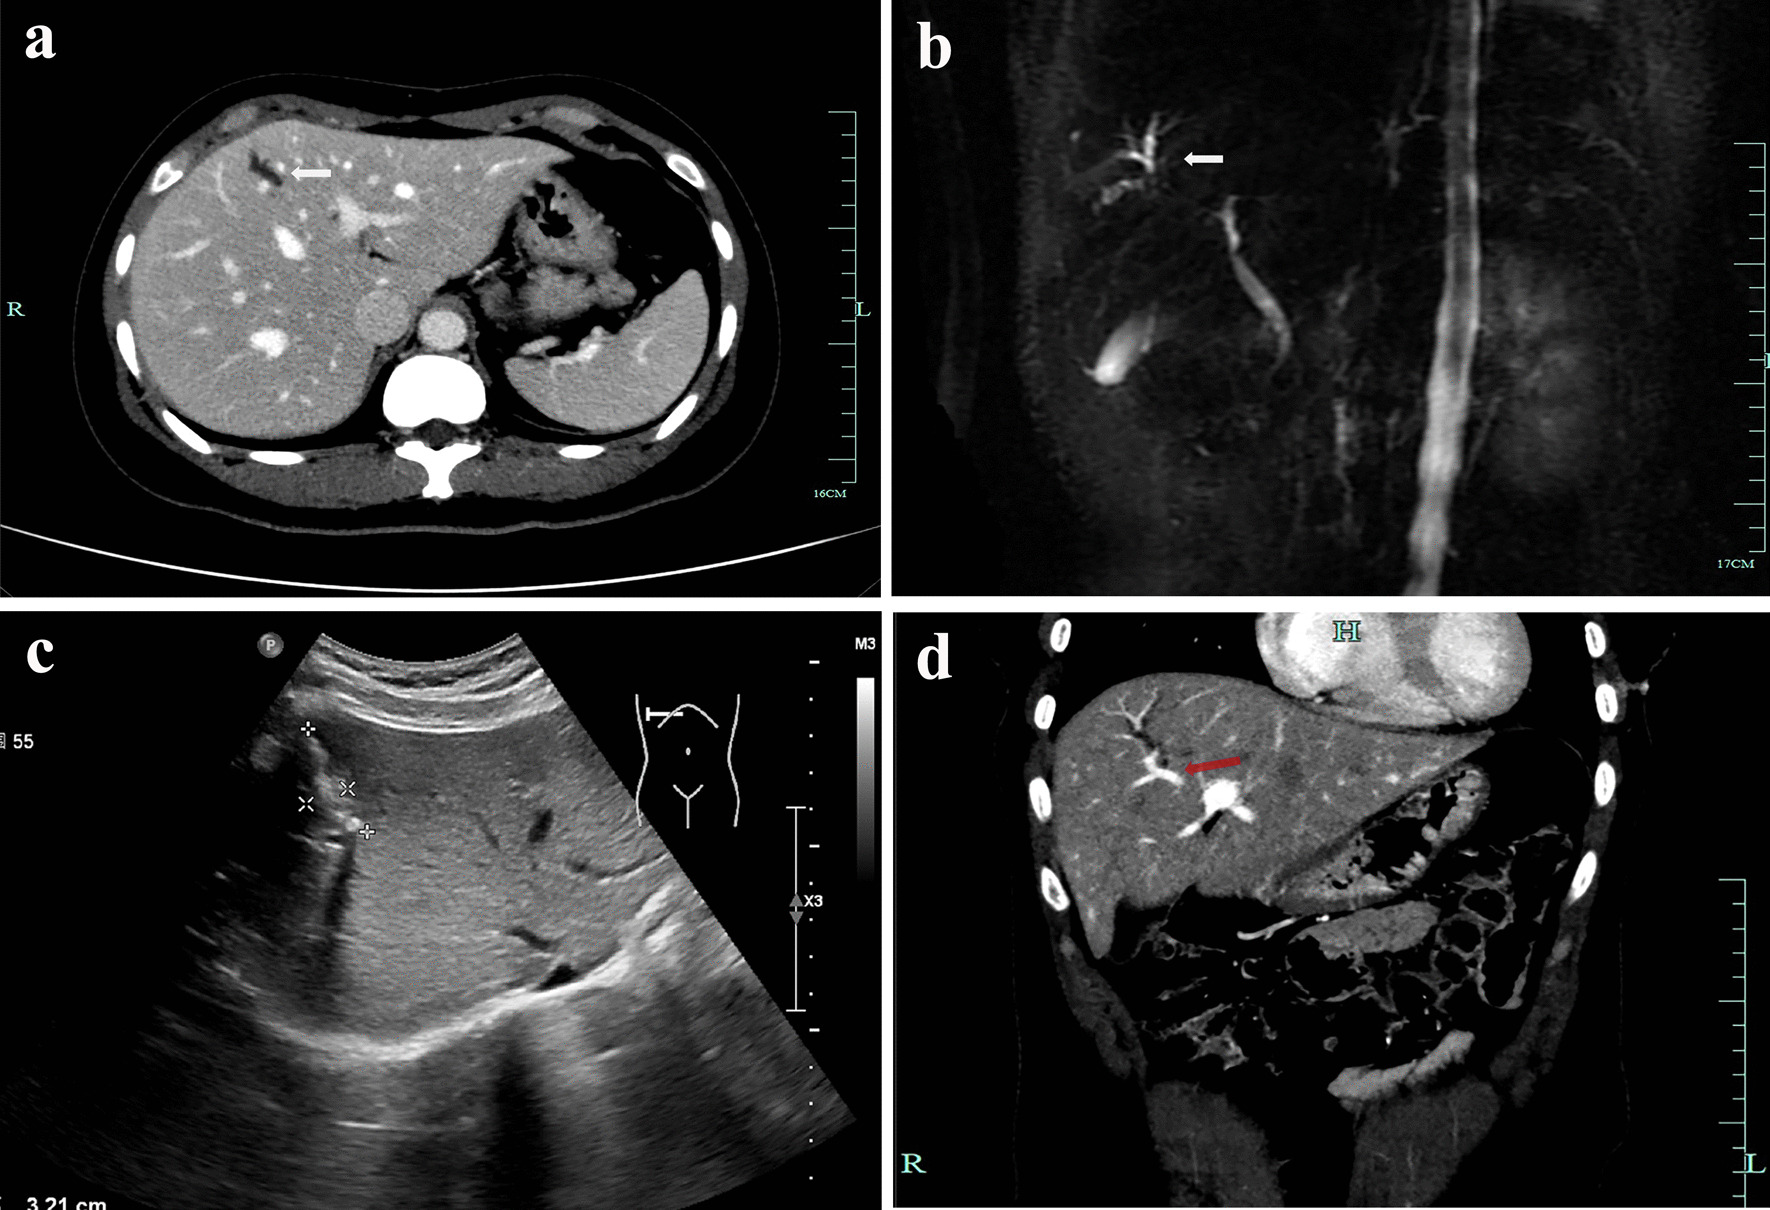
a Preoperative computed tomography imaging revealed localized intrahepatic bile duct dilatation in the superior region of hepatic S4 (white arrow); b Magnetic resonance cholangiopancreatography showed localized intrahepatic bile duct dilatation in the region of hepatic S4 (white arrow); c Ultrasound examination showed localized intrahepatic bile duct dilatation with cholangiolithiasis in the region of hepatic S4 (specific tag); d Computed tomographic angiography indicated the portal vein branch of the target hepatic segment (red arrow)
